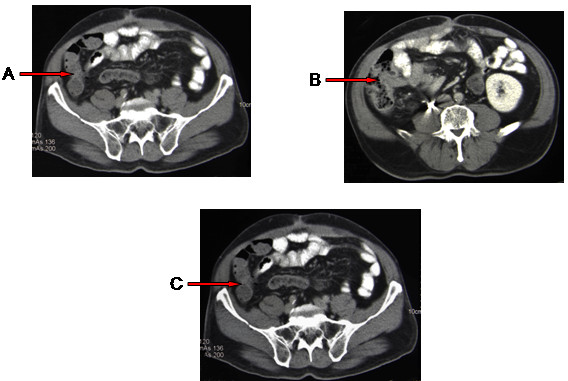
A-C) Computed tomography scan of abdomen with contrast shows normal appearing small bowel and colon during the asymptomatic phase.
